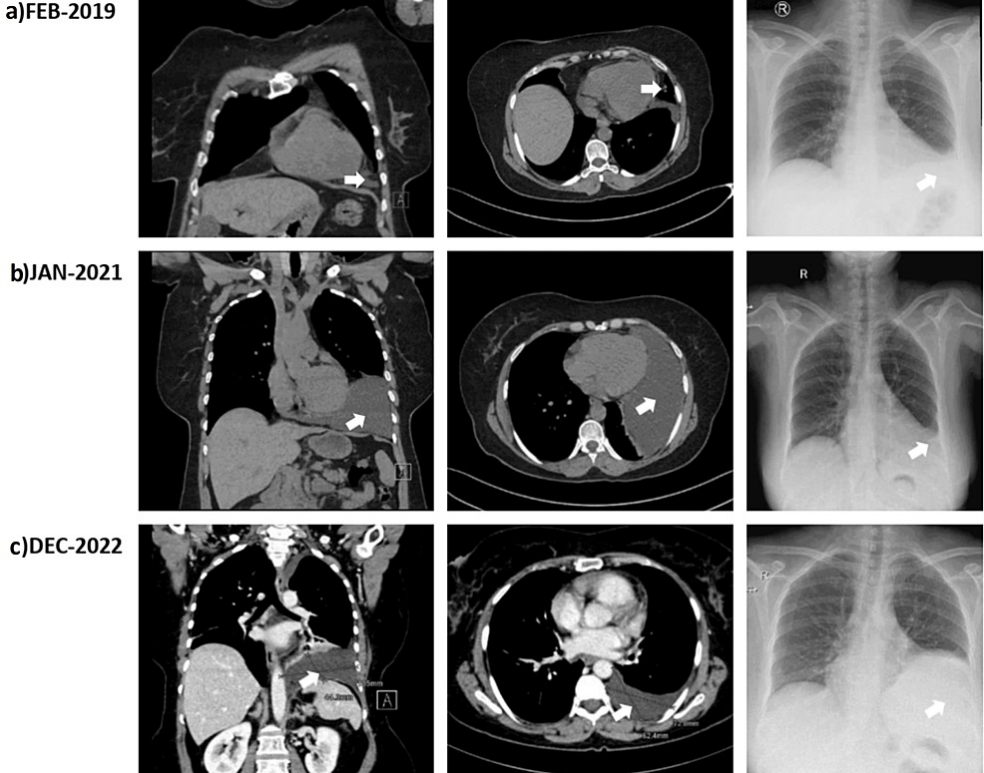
Serial CT scans and chest x-ray findings of left-sided unilateral low attenuating opacity changing size and shape, mimicking the appearance of a pleural effusion. CT: computed tomography The left column shows the coronal view of the CT The middle column shows the transverse view of the CT The right column shows the x-ray images (a) February 2019: CT chest done without contrast, (b) January 2021: CT chest done without contrast, (c) December 2022: CT chest and abdomen w/contrast.
